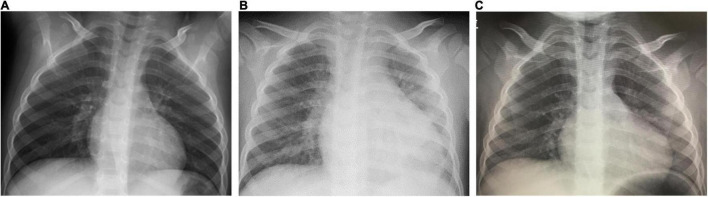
Chest X-ray findings. Chest X-ray on the day 3 [A, cardio thoracic ratio (CTR) 46%]; on the day 8 (B, CTR 62%); on the day 15 (C, CTR 53%).
